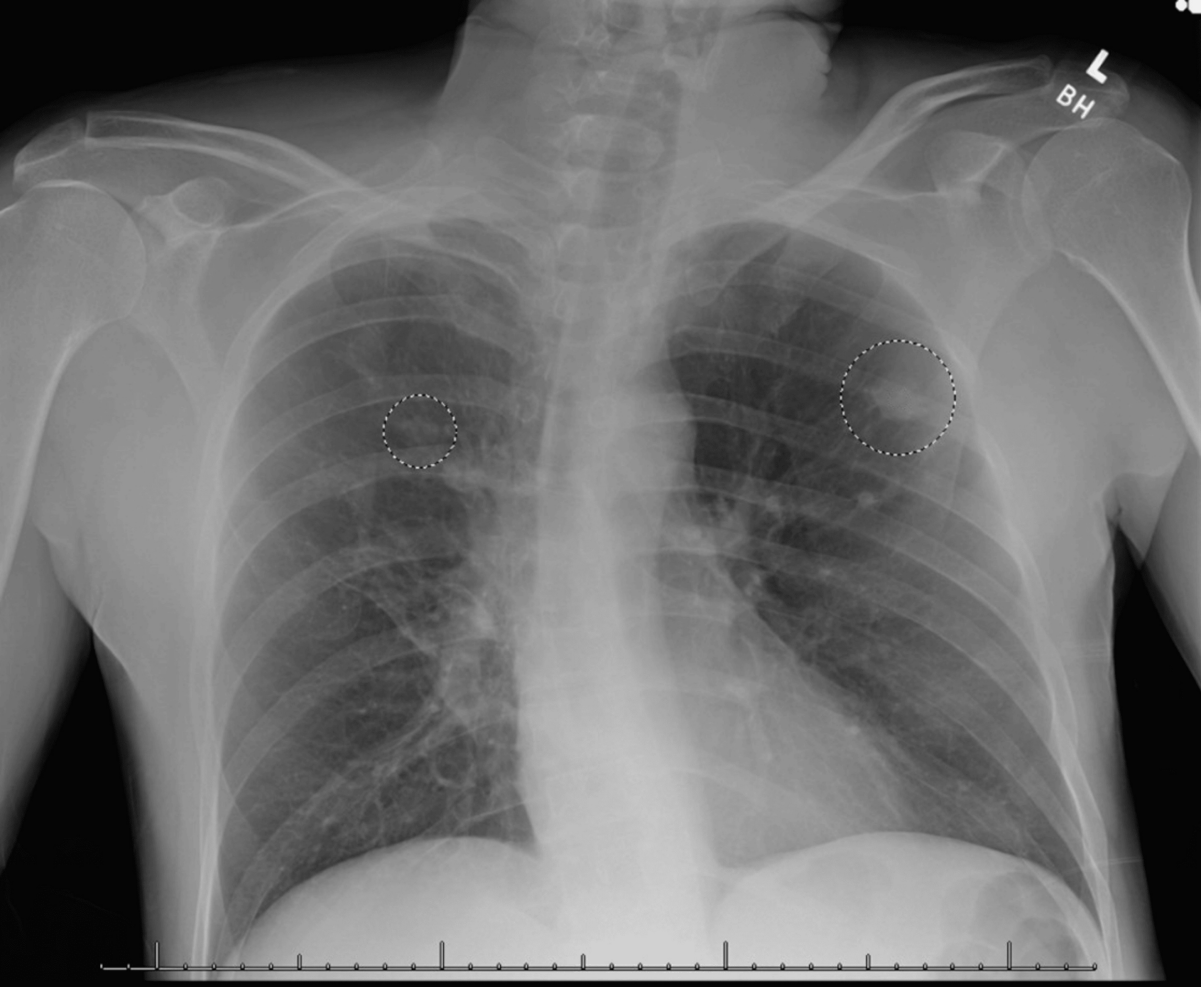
Chest X-ray Note the bilateral pulmonary nodules (circles) measuring 2.5 cm in the right upper lobe and 1.5 cm in the left upper lobe.
